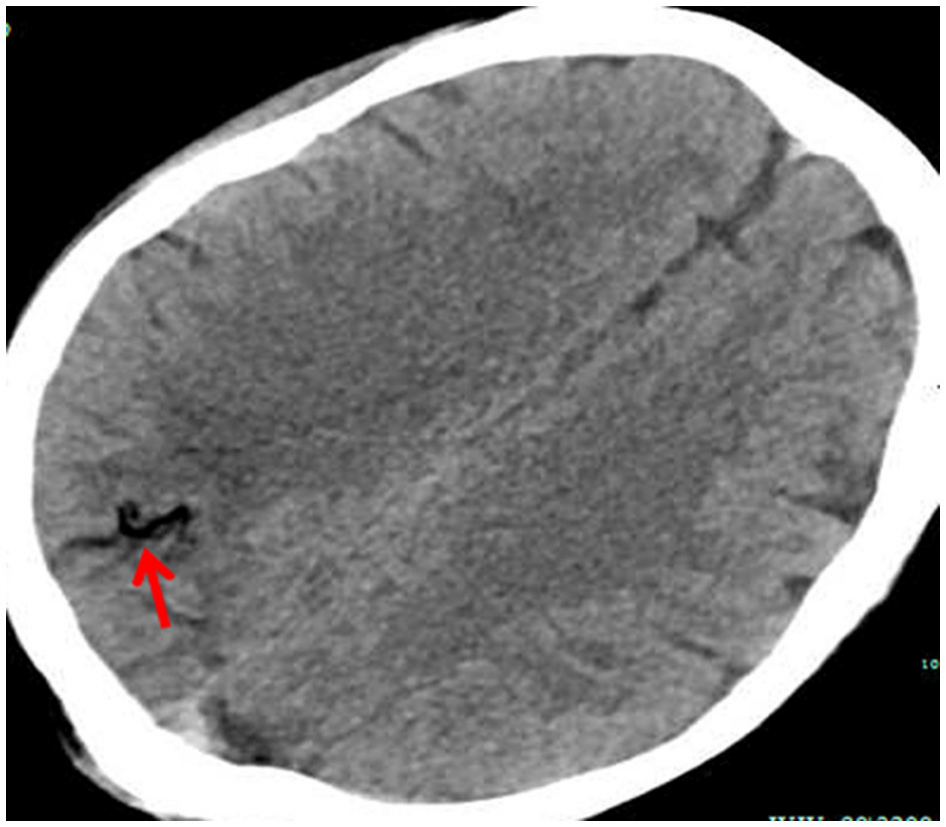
A brain CT scan showed multiple low densities in the right occipital lobe (red arrow).
